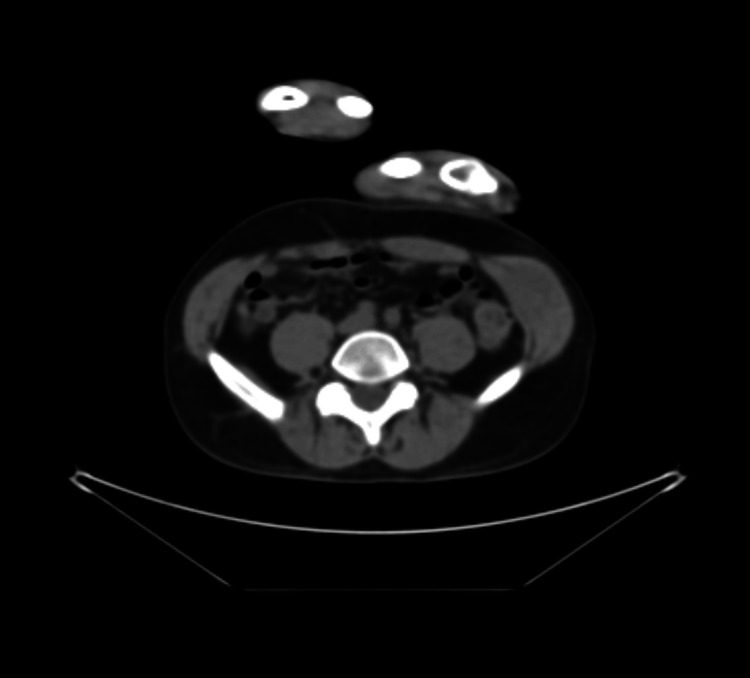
CT scan of the pelvis Asymmetry in size between the left and right internal oblique muscles of the abdominal wall due to an intramuscular mass in the left.
